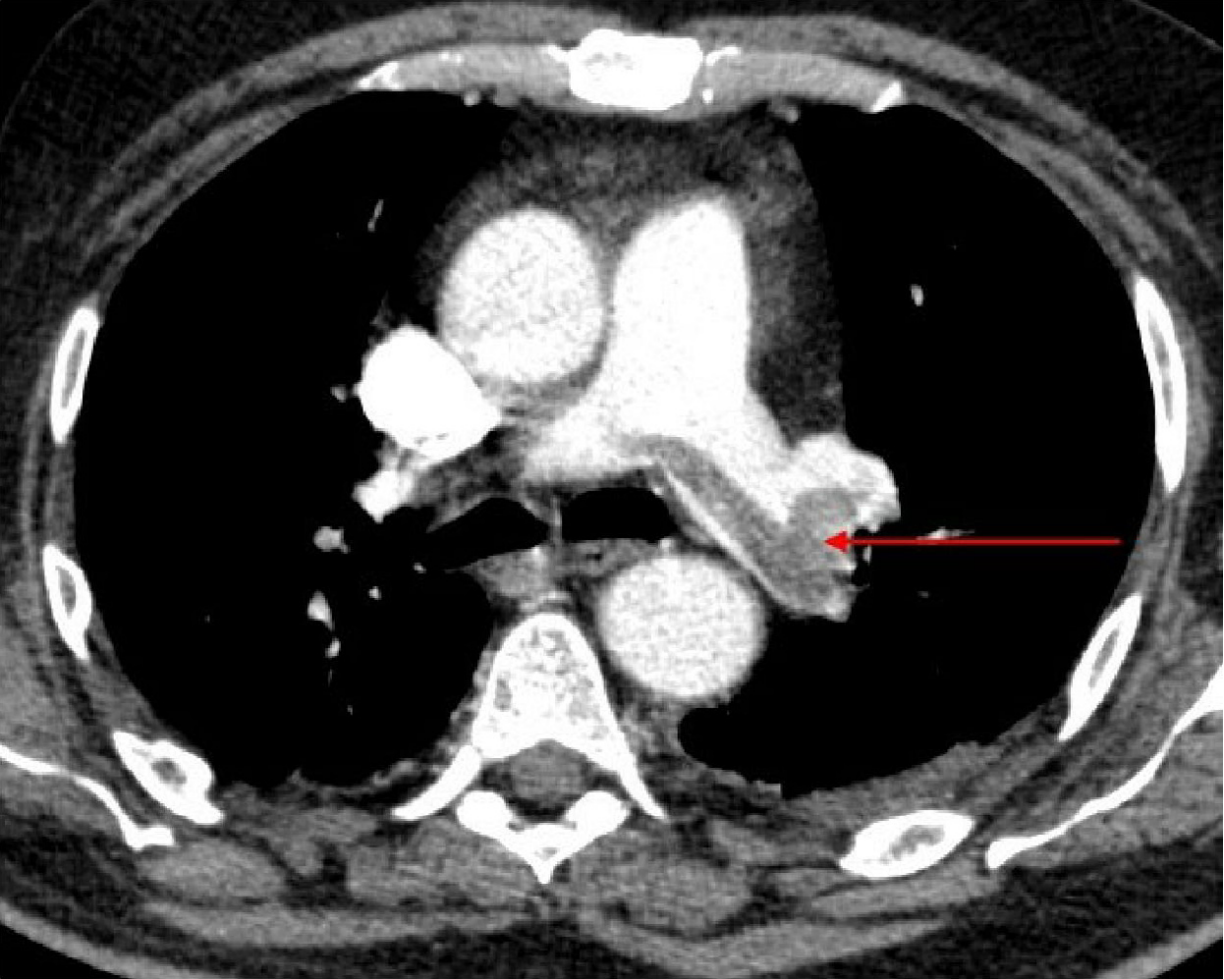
Computed tomography image showing pulmonary embolism.
